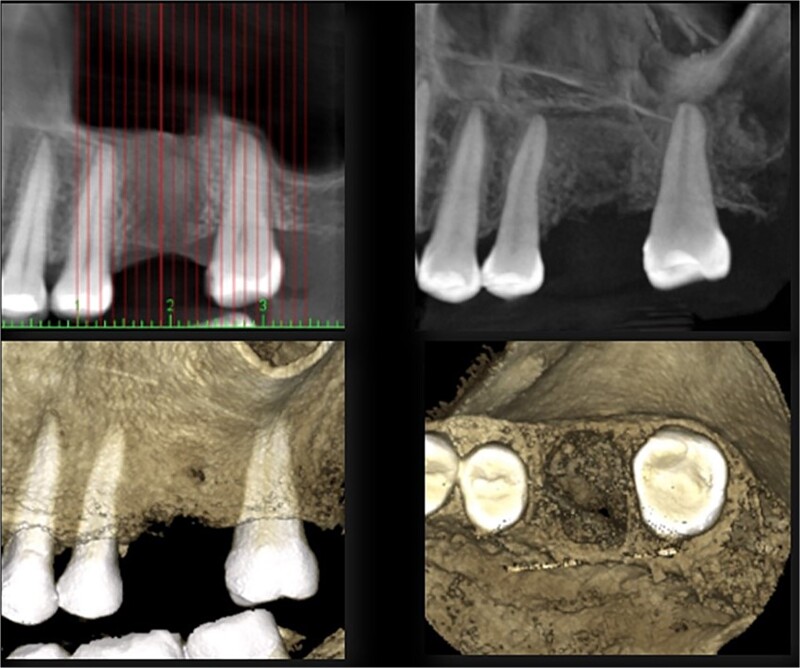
CBCT 3 months after surgery.
